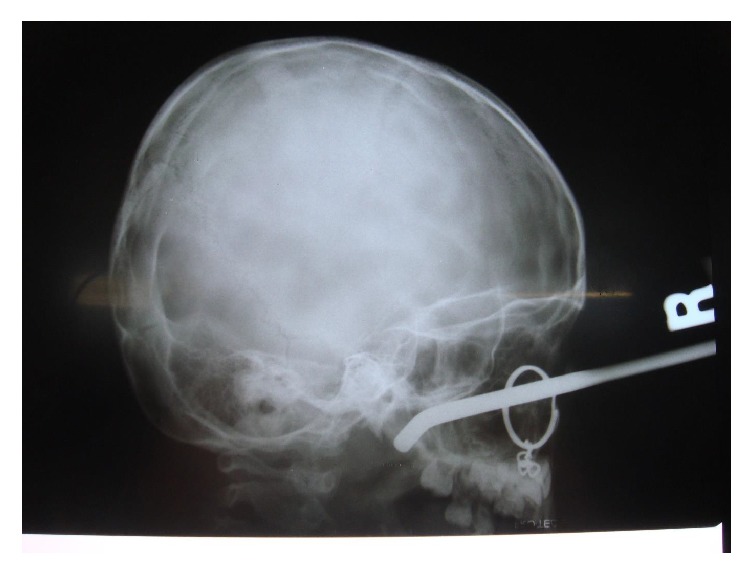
Preoperative X-ray of the skull lateral view, showing the Allen key in the left maxillary sinus to nasopharynx.
